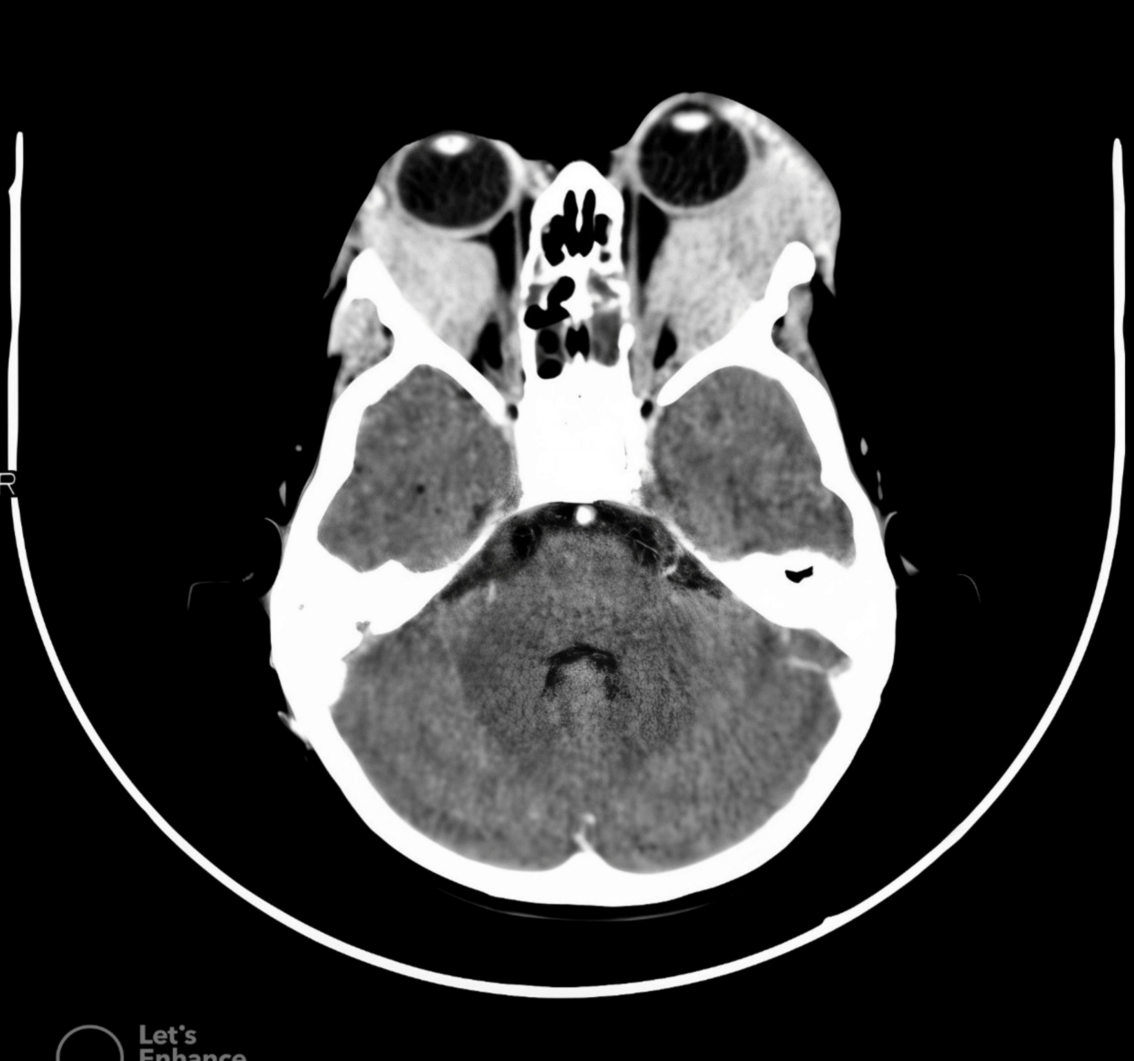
Axial CT Scan of the Orbits Showing Extensive Bilateral Retro-Orbital Masses; The Left Orbit Exhibits Severe Proptosis, and Orbital Wall Erosion Extends Inferiorly and Temporally, Demonstrating the Involvement of the Lacrimal Gland and Extraocular Muscles.
